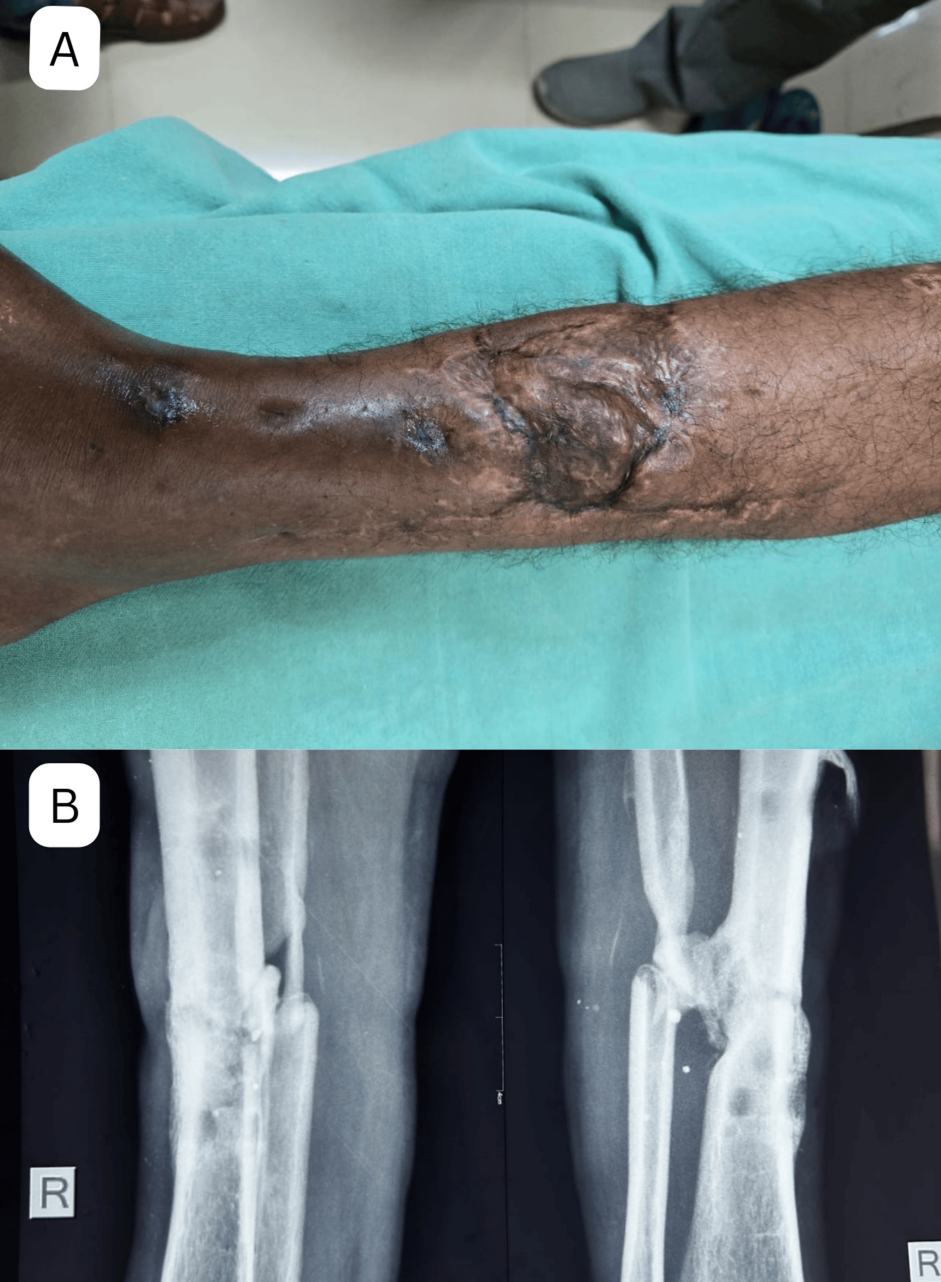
(A) Flap cover at seven months after removal of external fixator. (B) Anteroposterior and lateral radiograph at seven months since definitive procedure follow-up (nine months since injury) showing union of tibia, incorporation of bone graft with no sign of active infection. These images correspond to Case 1 from the study.
